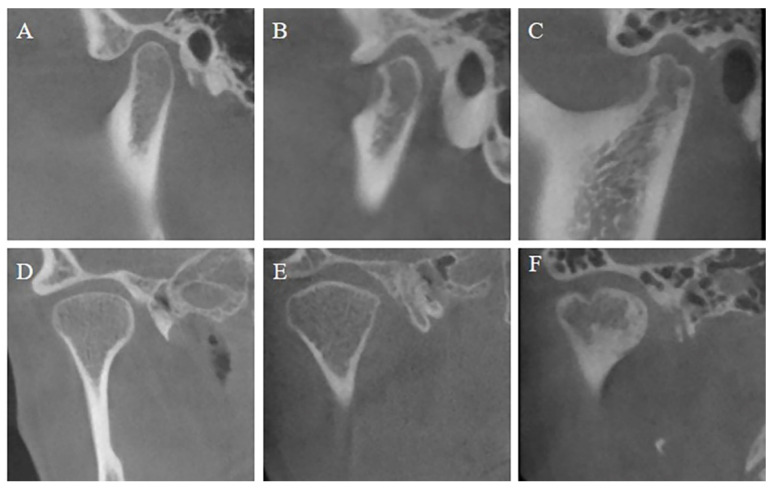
CBCT images of representative examples of condylar bony changes. (A) Sagittal view of normal condyle; (B) sagittal view of condyle with articular surface flattening; (C) sagittal view of condylar bony deformation; (D) coronal view of normal condyle; (E) coronal view of condyle with articular surface flattening; (F) coronal view of condylar bony deformation.
